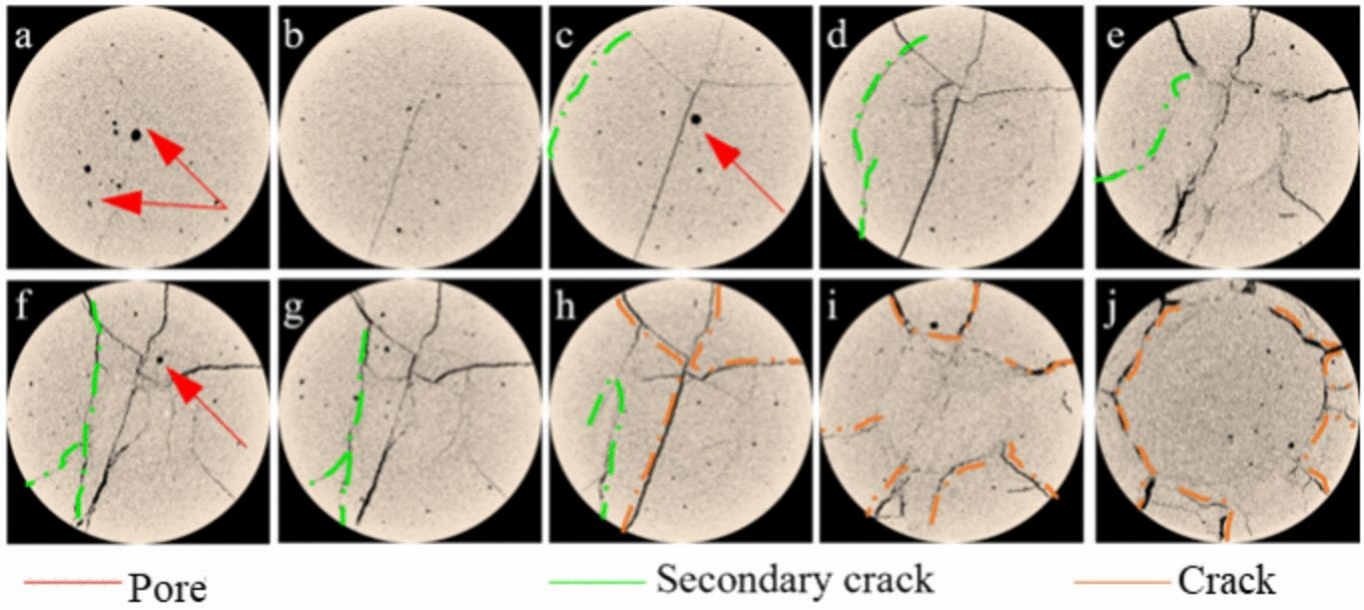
Investigation of X_2_Y_1_ failure modes at various heights from the CT system: (a)0 mm ~ (j) 100 mm.
